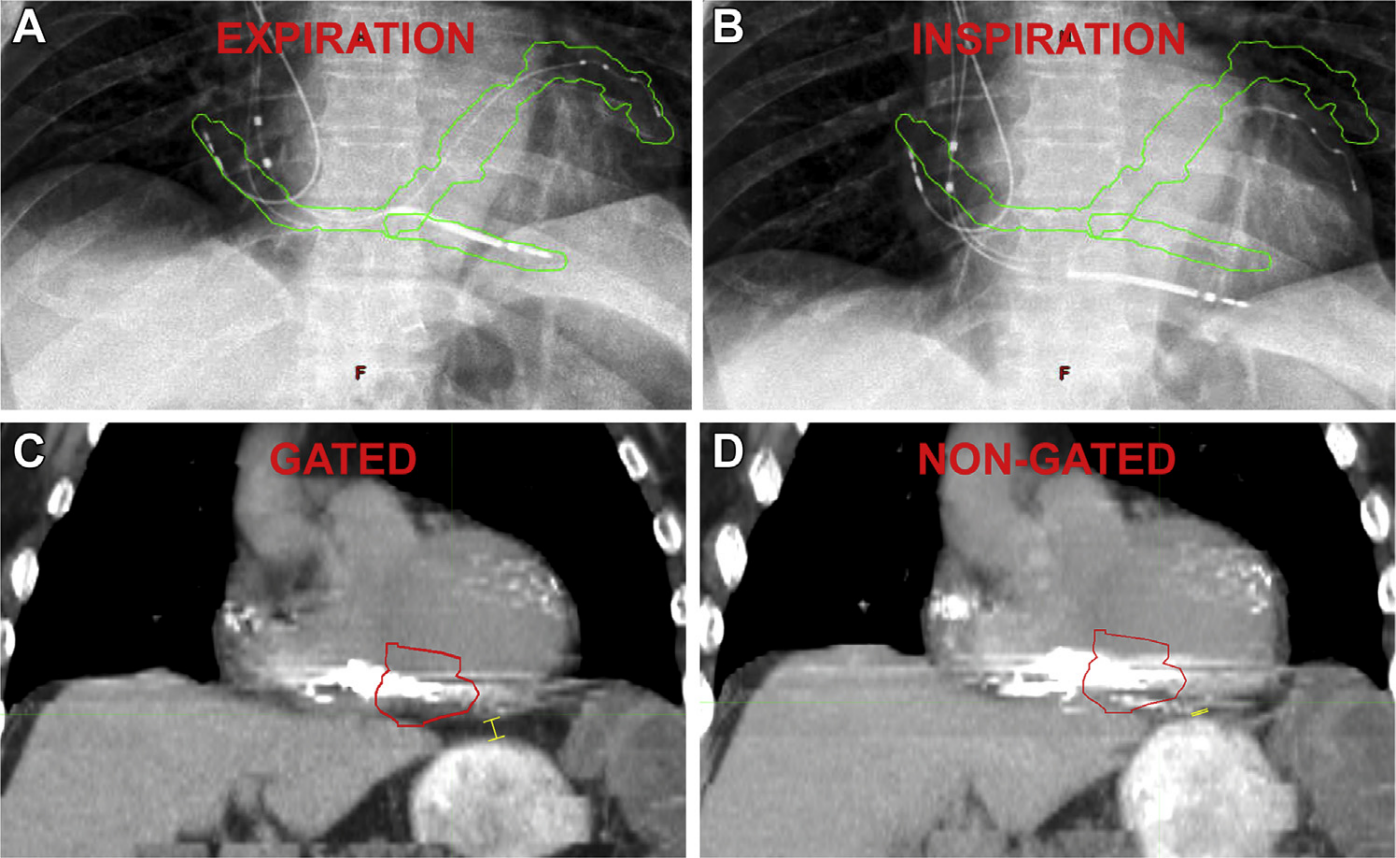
Respiratory-gated delivery of radiation. Example of patient 2, in whom respiratory-gated delivery was performed (see Supplemental Video 1). Fluoroscopy shows displacement of the implantable cardioverter-defibrillator (ICD) lead up to 2.2 cm during the respiratory cycle. The beam is turned on during expiration and off during inspiration, with the respiratory cycle tracked by an external optical tracking system. A, B: Proper timing of therapy is verified using intermittent fluoroscopy to confirm that the ICD lead is within the green contour during expiration (A) when beam is on and that the beam is off when the ICD lead is outside of the green contour during inspiration (B). C: On the gated expiratory phase 4-dimensional computed tomography study (4DCT), the inferior wall and ICD lead are clearly separated from the stomach (0.8 cm distance, *yellow marker*). D: On the nongated 4DCT, the inferior wall VT target (red planned treatment volume contour) and ICD lead are shown overlying the contrast-enhanced stomach (*yellow marker*).
